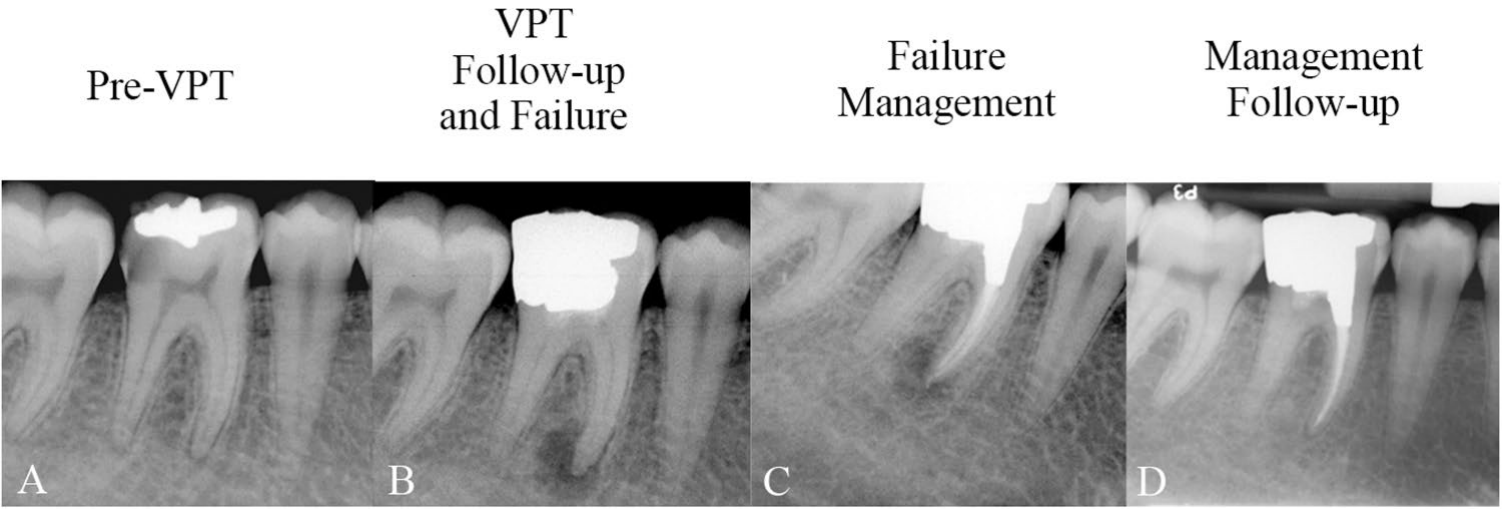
(A) Carious lesion on the right lower first molar of a 29-year-old man diagnosed with irreversible pulpitis/apical periodontitis, treated with full pulpotomy and amalgam filling; (B) Asymptomatic endodontic lesion detected during a periodic dental visit at 19-month follow-up, confirming failure; (C) Pulpectomy (selective RCT) and obturation of mesial canals and amalgam restoration of the involved tooth performed while the distal root remained untouched; (D) Complete healing of the periapical lesion noted at 42-month follow-up, with the tooth remaining functional, confirming success.
